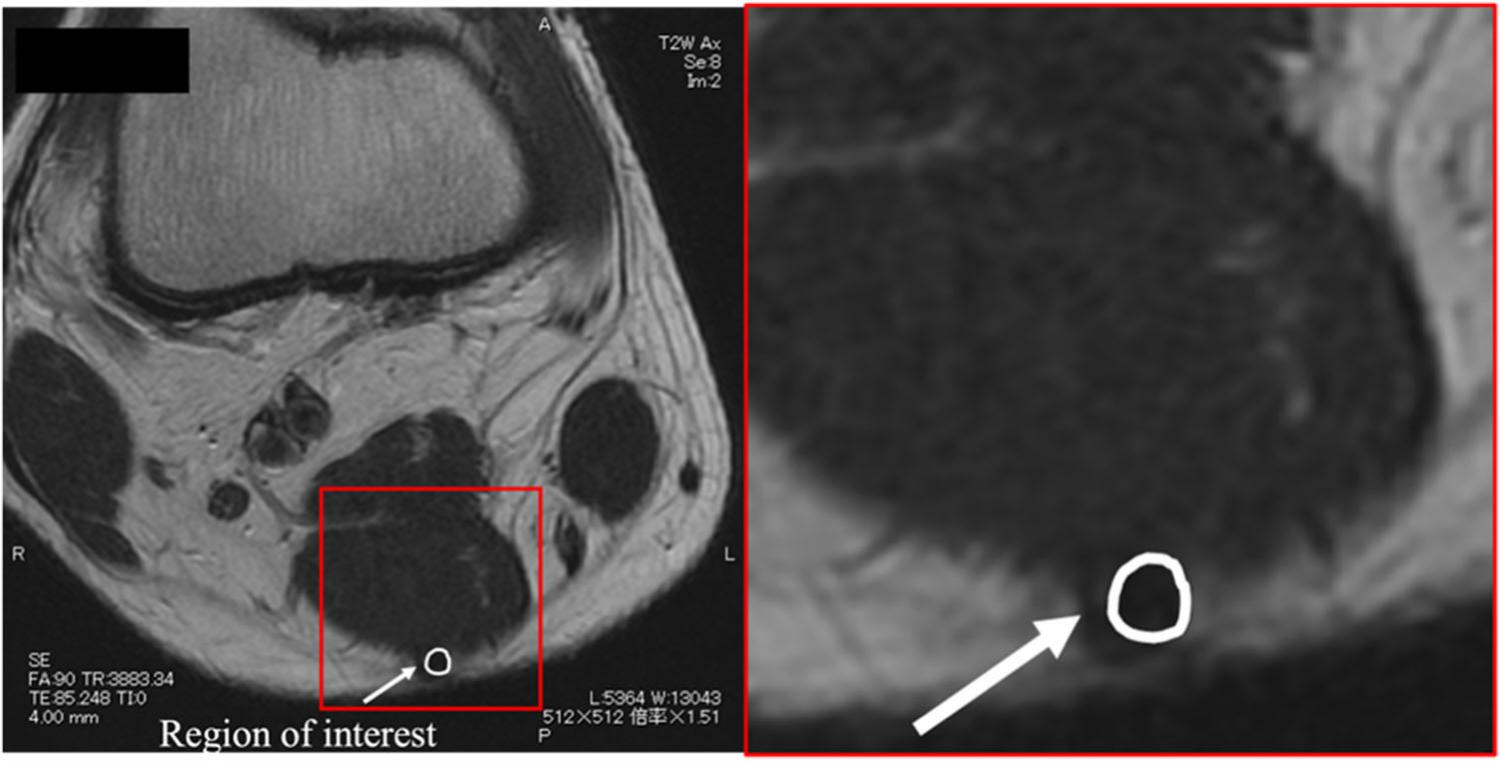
Measurement of the cross‐sectional area of the semitendinosus tendon using magnetic resonance imaging T2 weighted images. Measurements were calculated using a polygonal region of interest at a position 8 cm proximal to the tibial attachment of the semitendinosus tendon.
